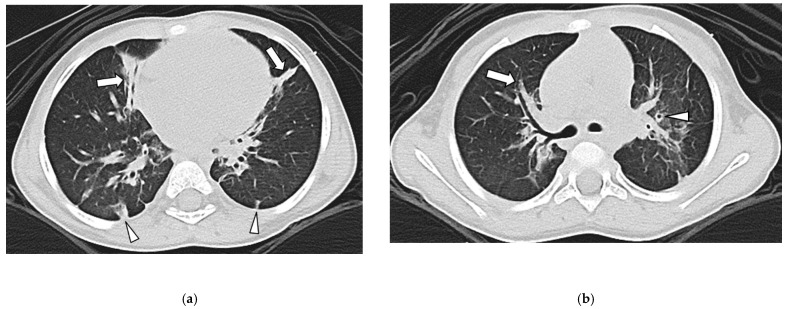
Three-year-old girl with prematurity-related lung disease. (a): Axial lung window CT image shows triangular/linear plaque-like opacity (arrows) in both lungs. Pleural based atelectasis (arrowheads) is also seen. However, no pleural thickening is noted. (b): Axial lung window CT image demonstrates bronchial wall thickening (arrowhead) and bronchiectasis (arrow).
